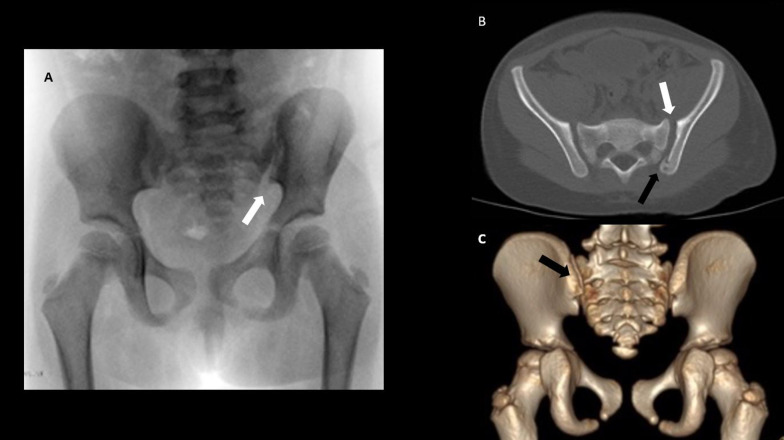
A type 1, Risser growth plate injury (RGPI). Notice the minimally displaced SI Joint (*arrow*) On the X rays the left hemipelvis appears intra-rotated respect to the right side. The CT scan shows a fracture line involving the posterior iliac spines. The three-dimensional (3D) image shows a fracture line that runs through the posterior iliac crest
